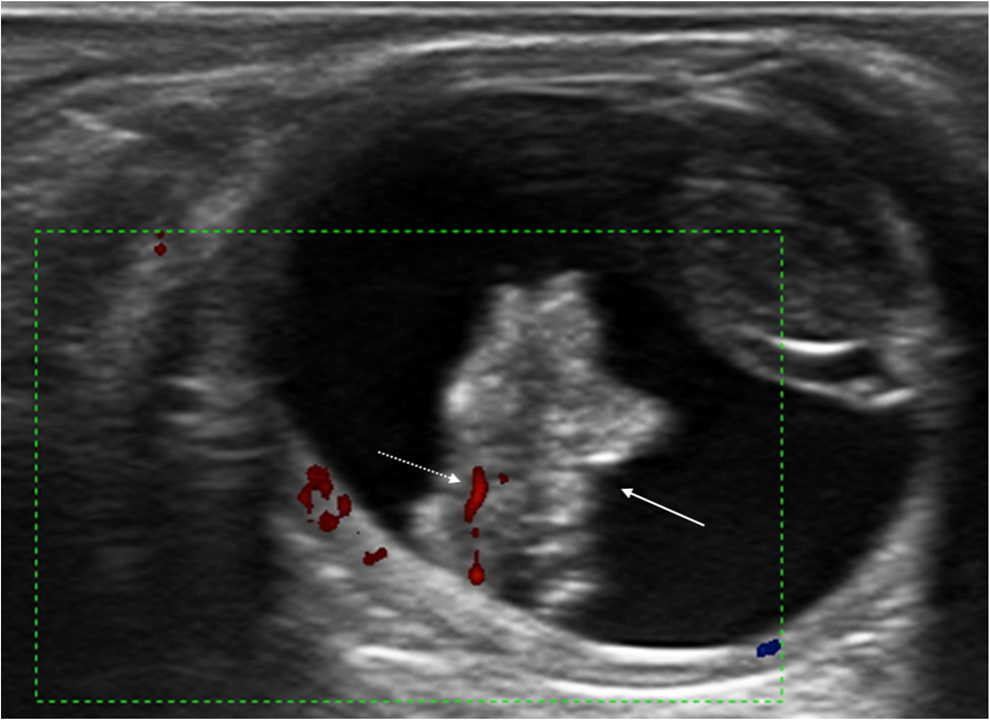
Ocular US in a 25-year-old woman with trisomy 21 and persistent hyperplastic primary vitreous. Axial US image shows a right eye that is smaller than normal, with a linear echogenic tract extending from the posterior surface of the lens to the posterior ocular wall representing the fibrovascular remnant (*arrow*). Colour Doppler shows arterial blood flow within the band that corresponds to persistence of the hyaloid artery (*dotted arrow*). Note also the cataract
